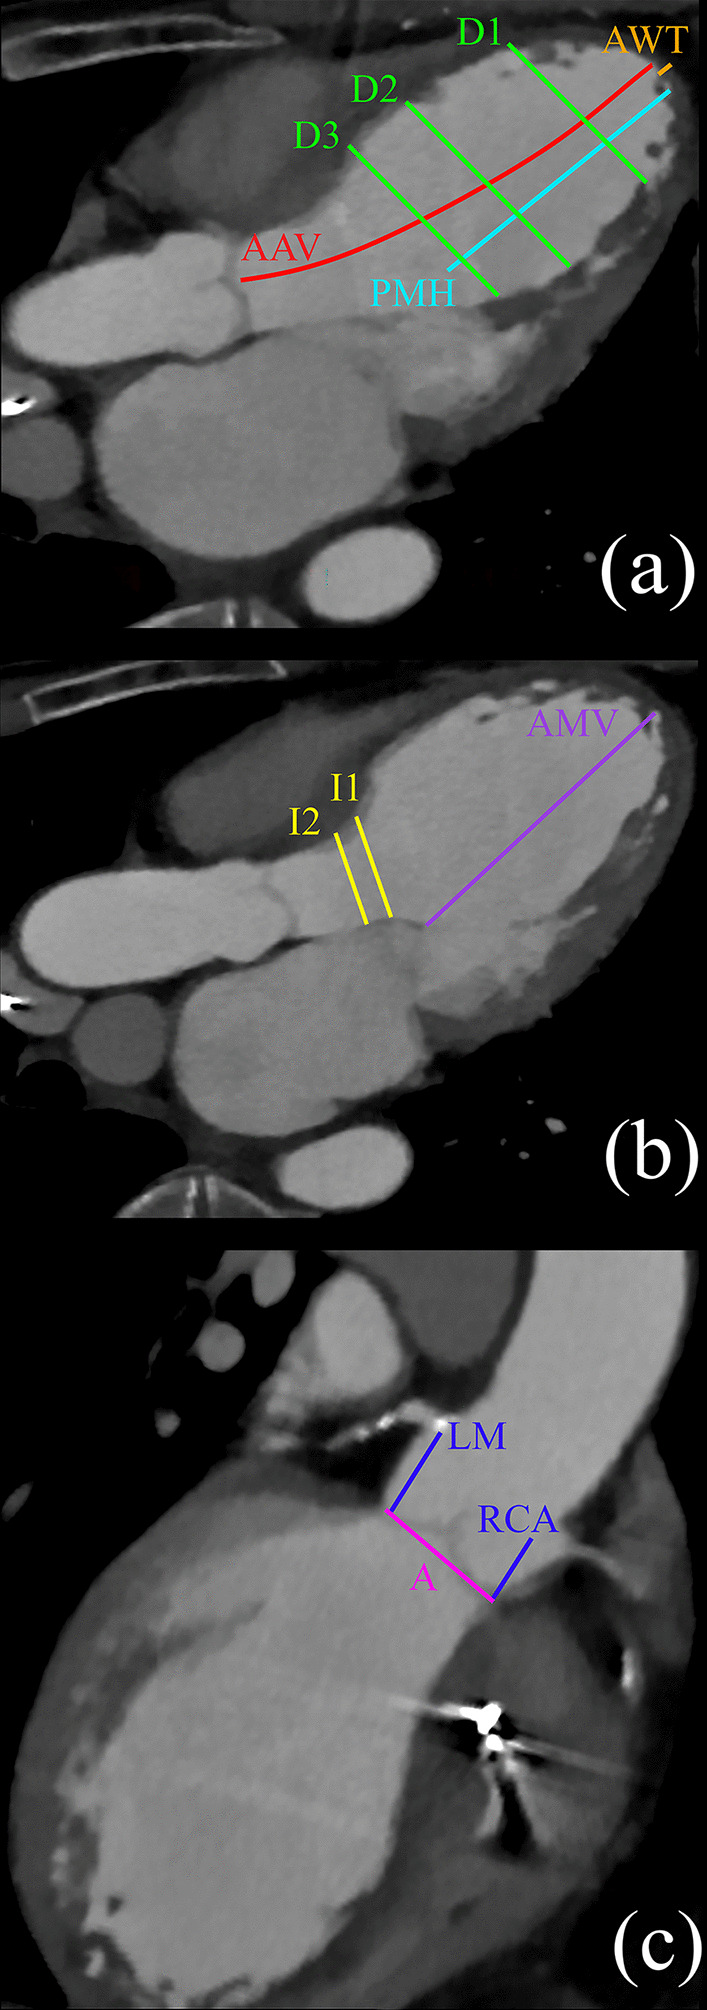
Studied cardiac CT images in diastole showing our measurement. In (a) and (b), the anterior mitral leaflet can be seen in an opened position. In (c), measures of the coronary artery openings and the annulus are visualized. The measured dimensions are seen with different colors and labeled appropriately with their abbreviation given detailed in the text
